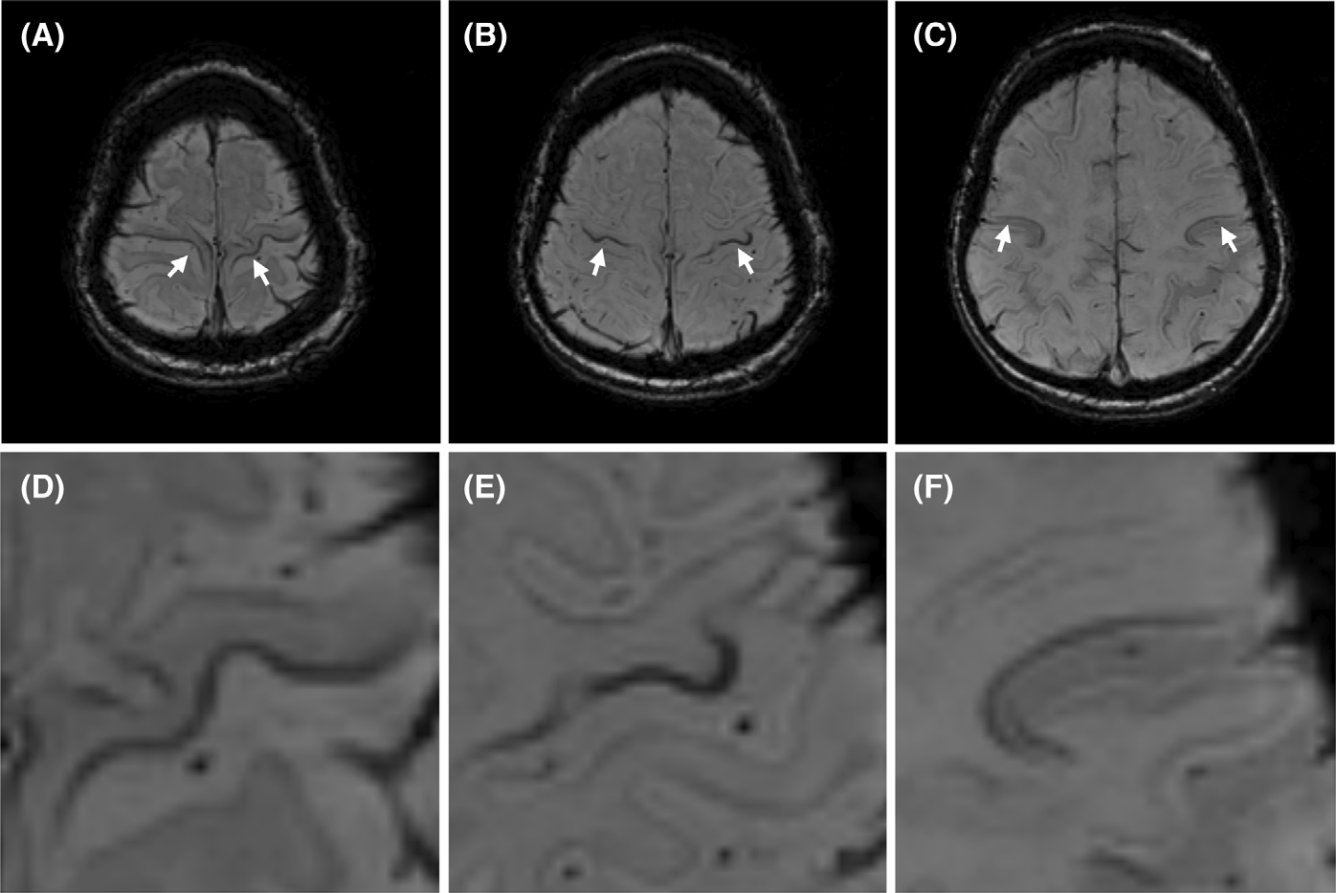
Motor band sign topographical classification. Anatomical distribution of primary motor cortex hypointensities, the motor band sign, on axial susceptibility‐weighted brain MRI in a 72‐year‐old female patient with dysarthria and mild motor weakness in the upper and lower extremities. D–F showing magnifications of A–C, respectively. There was marked hypointensities in the medial (A and D), intermediate (B and E), and lateral (C and F) regions of the motor cortex.
